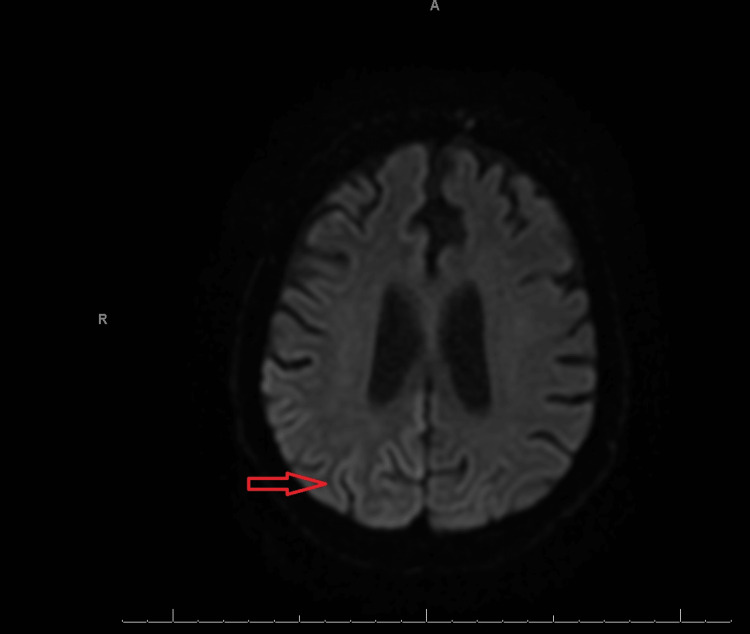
MRI of the brain (DWI) without contrast displaying areas of curvilinear hyperintense signal in the right temporo-occipital cortex (red arrow) Curvilinear hyperintense signal with cortical restricted diffusion is called cortical ribboning. DWI: Diffusion-weighted imaging.
